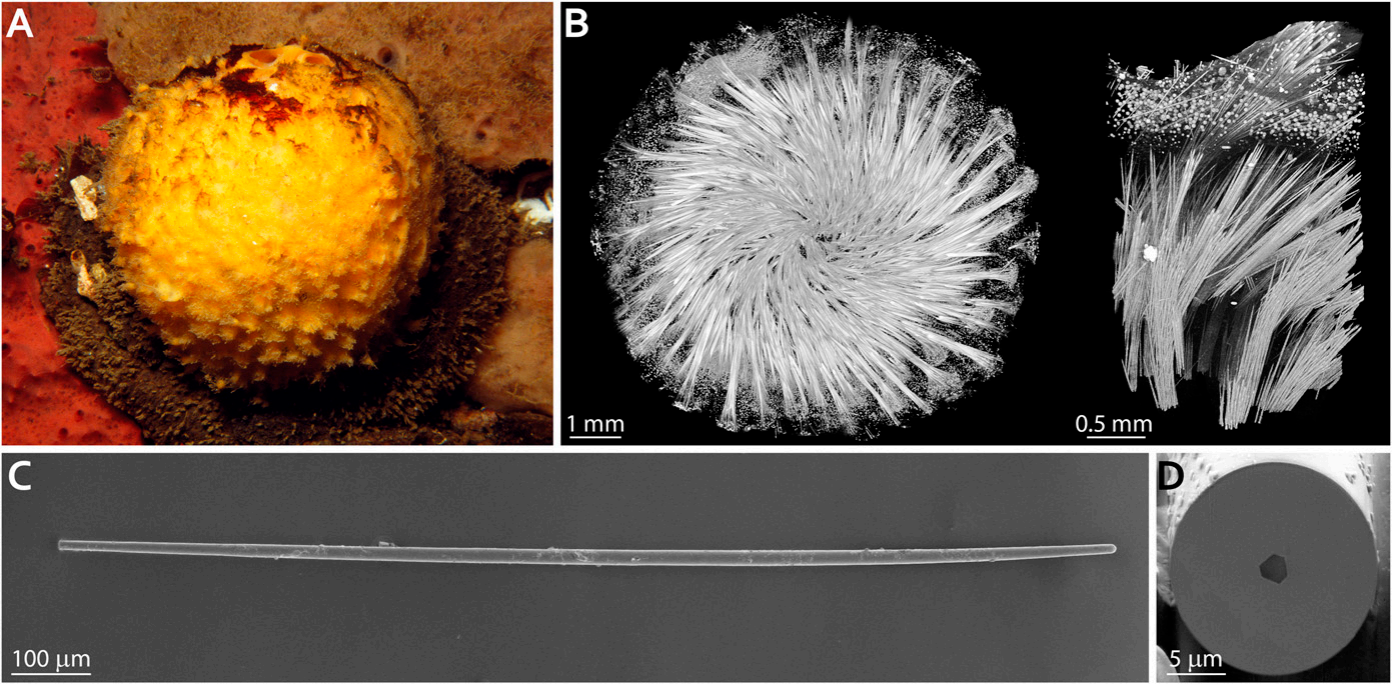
Skeletal elements of the demosponge *T. aurantium*. (*A*) The demosponge *T. aurantium*. (*B*) 3D visualization of the mineralized silica skeleton in *T. aurantium* obtained using X-ray microtomography. (*Left*) An entire sponge skeleton. (*Right*) A crop out of the cortical part of the sponge. (*C*) A single needle-like spicule (strongyloxea) extracted from the cortical region of *T. aurantium*. (*D*) A cross-section through the spicule in *C*, cut using FIB milling, demonstrating the presence of an axial filament going through the center of the spicule.
